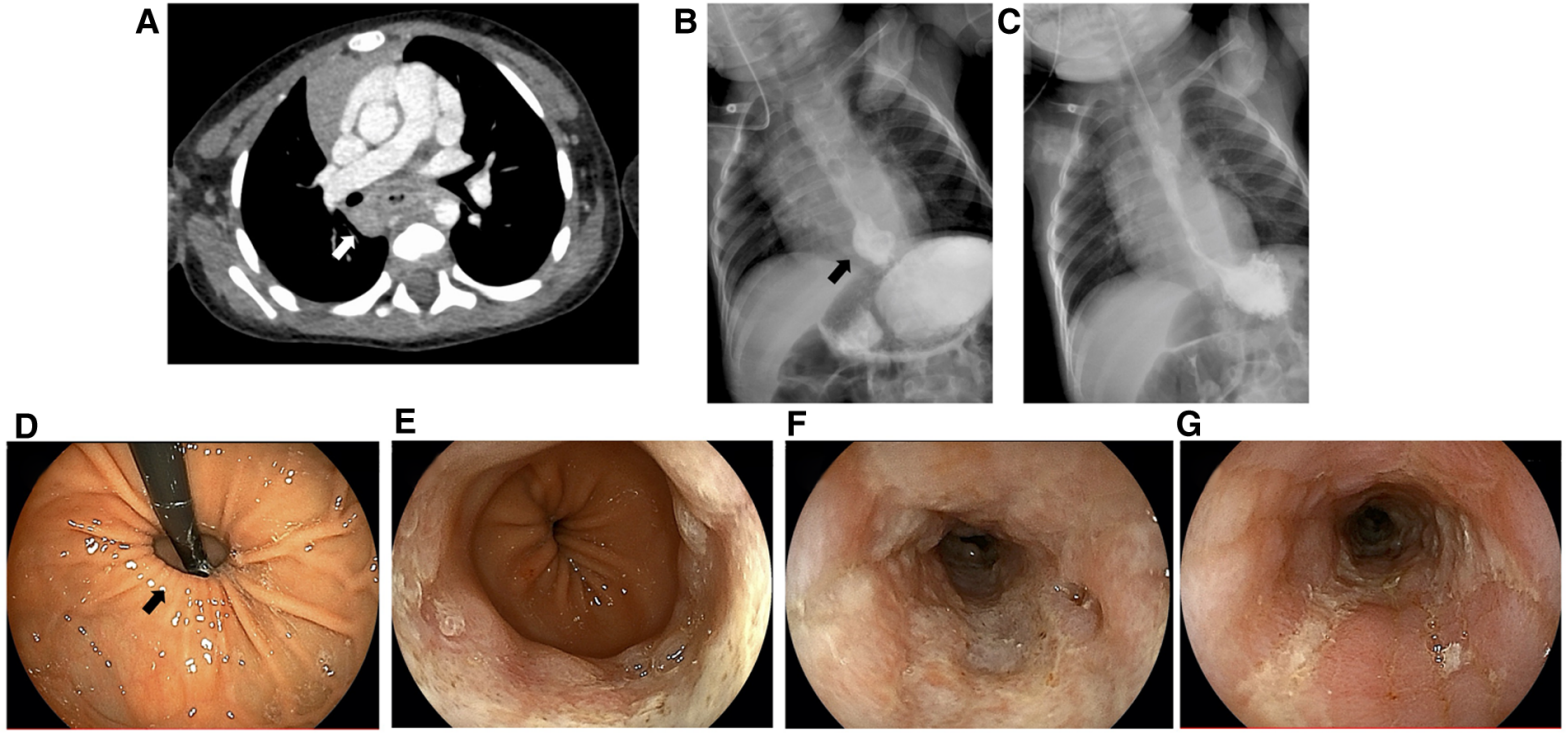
Abdominal computed tomography (A), upper gastrointestinal series (B,C), and esophagogastroduodenoscopy findings (D–G). White arrow, lymphadenopathy; black arrow, hiatal hernia.
